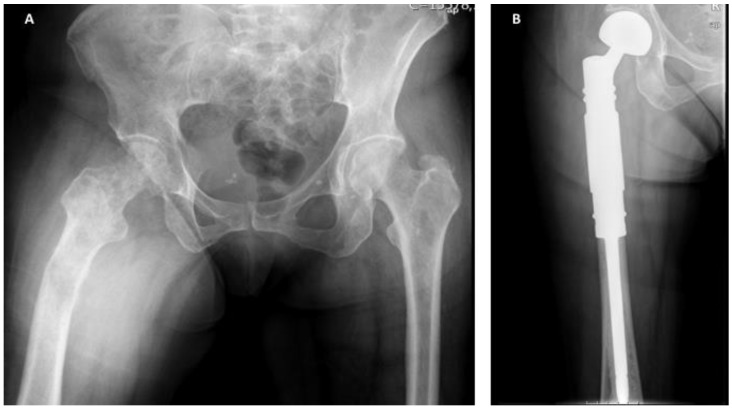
Extensive breast cancer bone metastasis in a female patient with multimetastatic bone disease: (A) anteroposterior pelvic radiograph showing a pathologic fracture in the right femur, not amenable for reconstruction due to extension of the metastatic bone disease, (B) immediate postoperative radiograph showing anteroposterior view of the right femur with a bipolar tumoral hip megaprosthesis, used to provide stability and pain relief. After surgery, the patient underwent local radiotherapy and bone-targeted agents.
